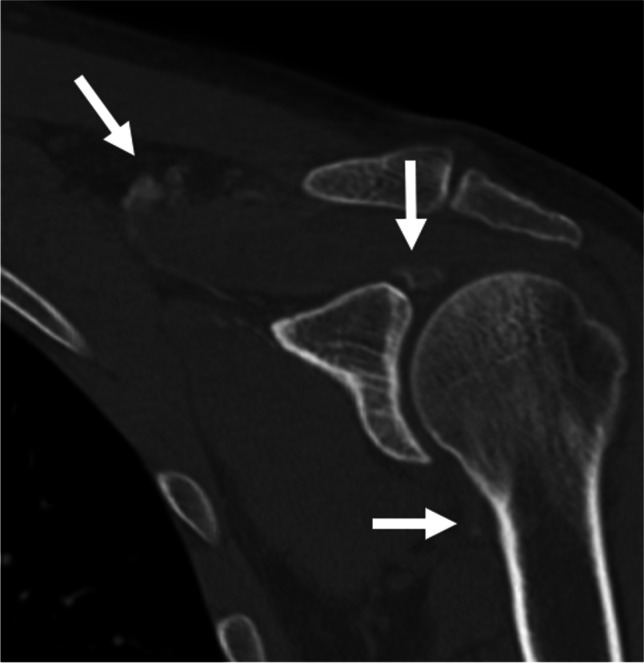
A 51-year-old male presented with a 1-week history of severe left shoulder pain after lifting weights at the gym. CT of the left shoulder with coronal reformations demonstrates a gross case of ACP, with capsular/pericapsular calcific deposits and extensive further calcification which tracks medially into the supraclavicular fat (arrows)
